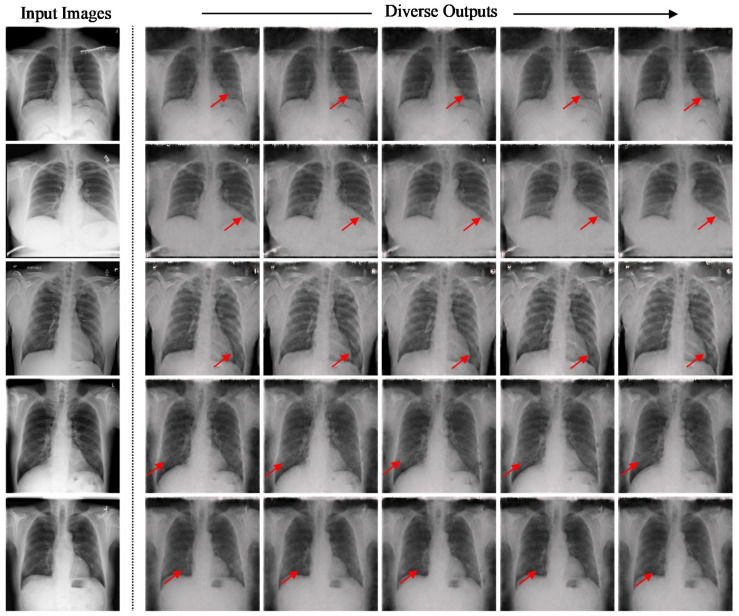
Examples of instance-diverse COVID-19 X-ray images generated from the sRD-GAN framework. The red arrows point to the region where the style attributes generated on the output images are different.
